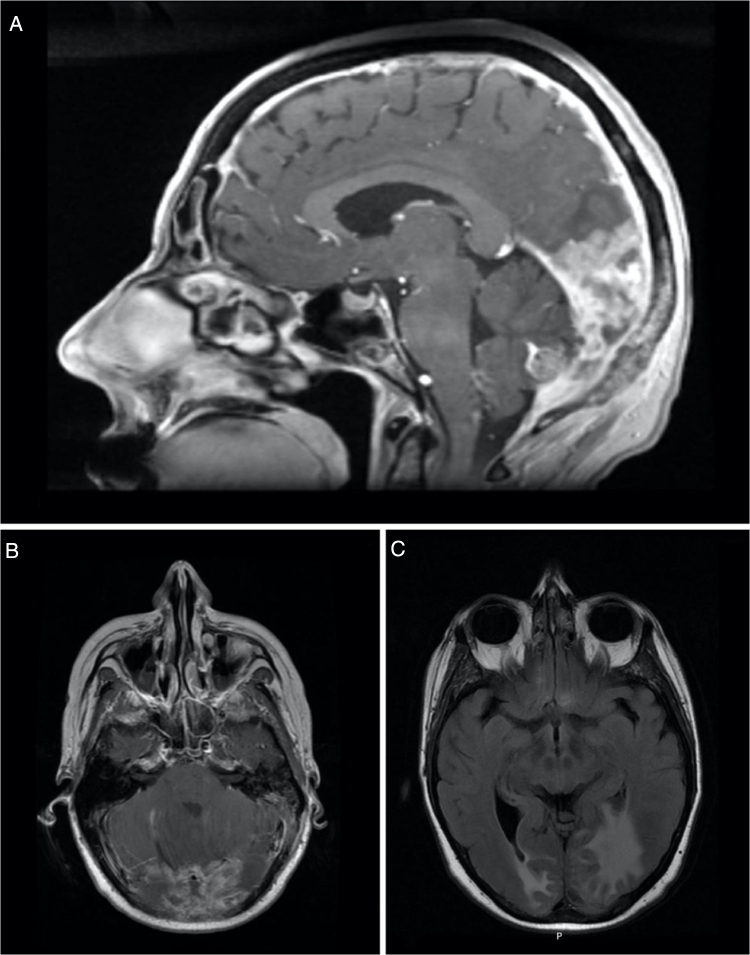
Sagital (A) and axial (B) contrast-enhanced T1-weighted MRI showing thickening and enhancement of the dura suggestive of pachymeningeal carcinomatosis (arrow). Axial (C) T2-FLAIR-weighted MRI showing vasogenic edema in the right occipital lobe and left occipito-temporal region.
